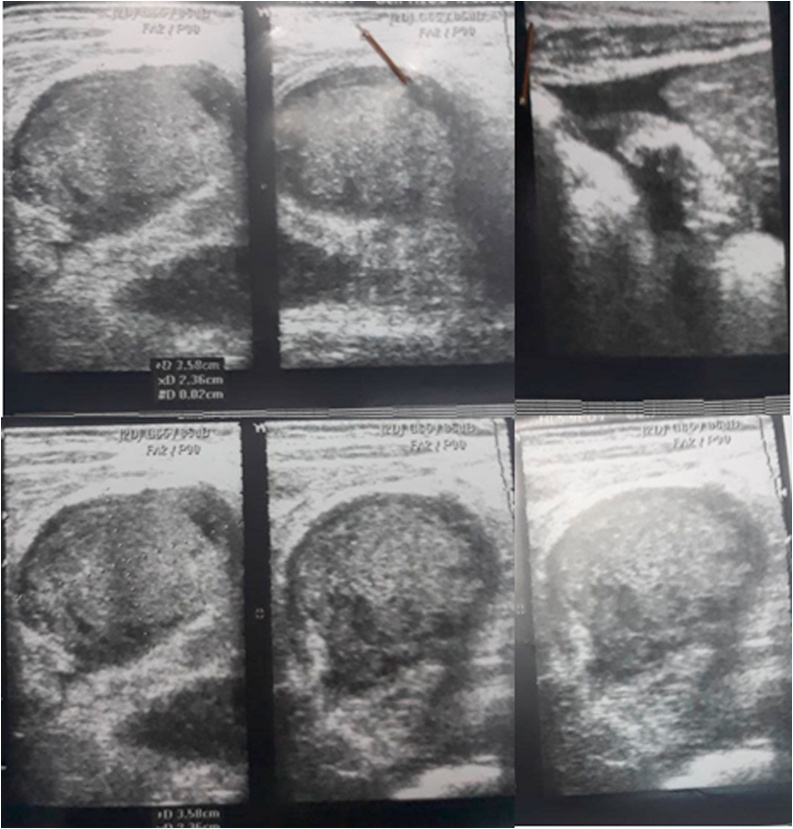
Ultrasonography of the oval mass of 2.5*3.5 cm which was suspected to be acute intussusception.
